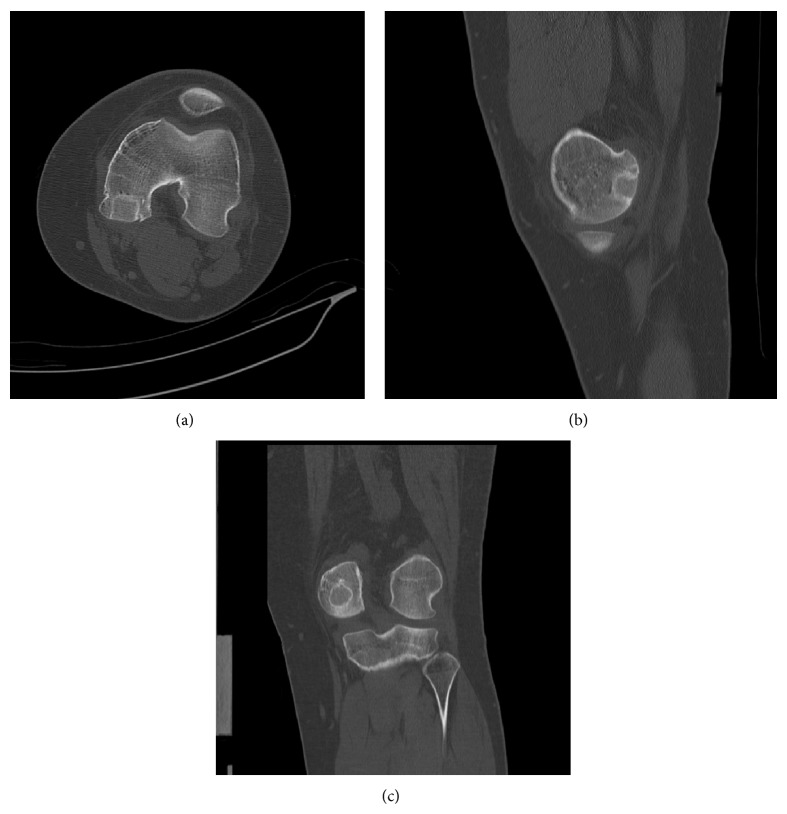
One-year postoperative axial (a), sagittal (b), and coronal (c) CT scan demonstrating osteochondral allograft incorporation and congruency of chondral surface. No evidence of local recurrence.
